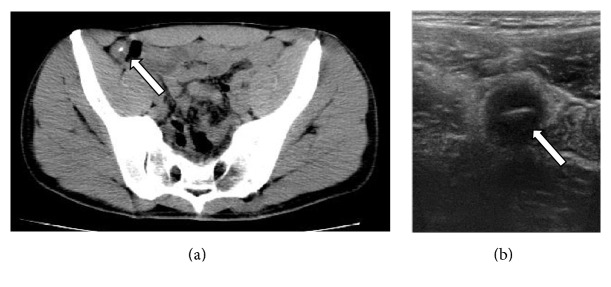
Axial images of MDCT (a) show a foreign body in a small bowel loop (white arrow), also detected in abdominal ultrasonography (b).
